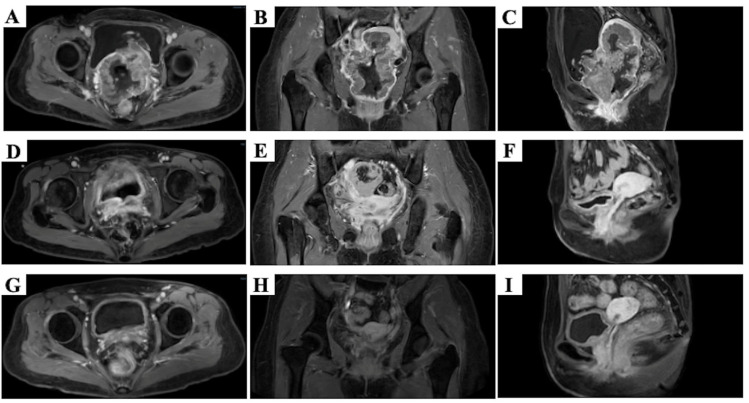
The images of pelvic MRI in an axial plane (A,D,G), in a coronal plane (B,E,H) and in a sagittal plane (C,F,I). Before treatment (A–C), after LRT and 2 cycles of induction chemotherapy (D–F) and 3 months after the completion of CCRT (G–I).
